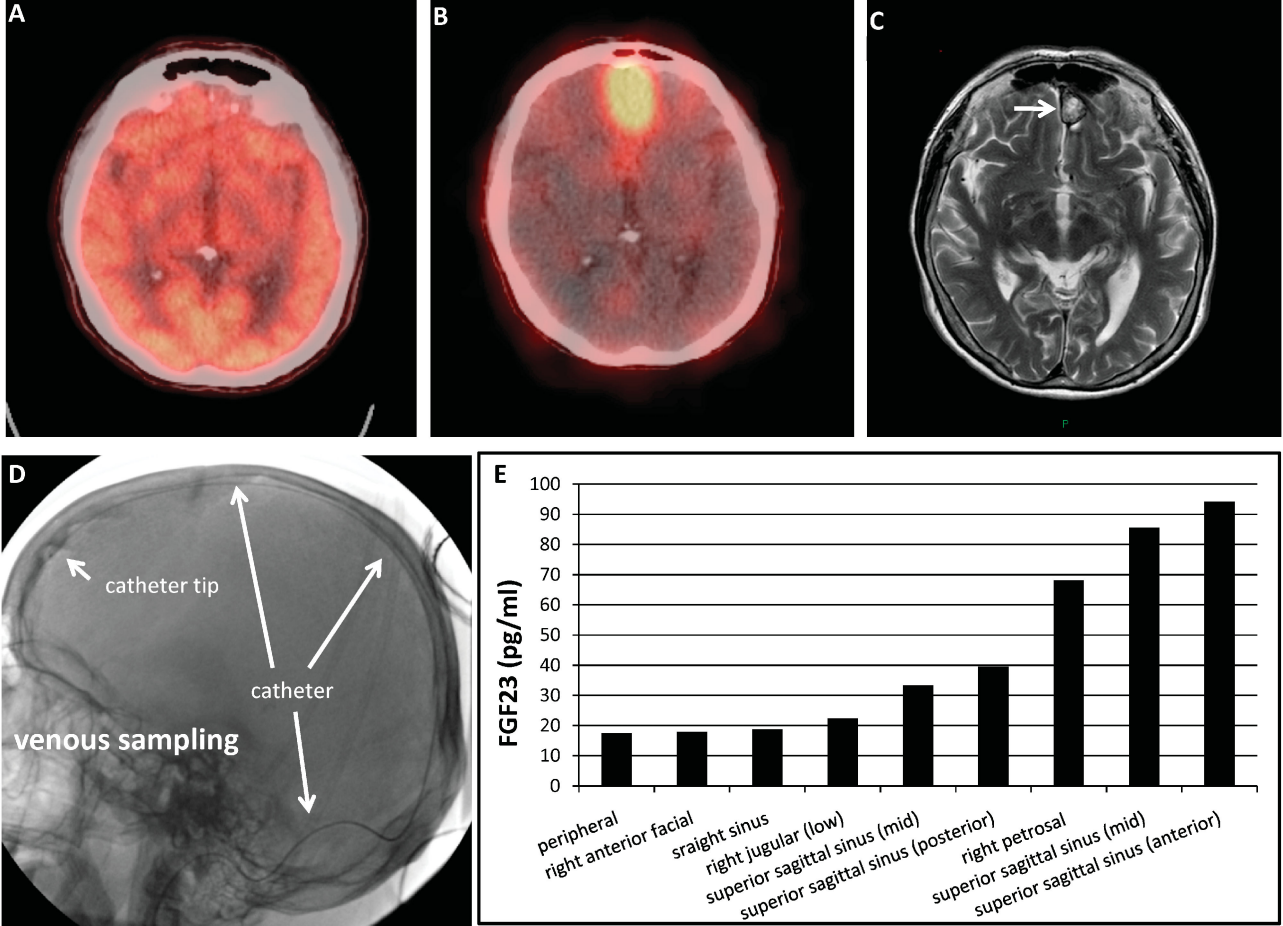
Example of a selective venous sampling that distinguished between conflicting imaging results. For a lesion that ultimately was found adjacent to the brain, FDG-PET/CT scan (*A*) was negative owing to the intense physiologic uptake of FDG by the brain. The pentetreotide/CT scan was positive (*B*), but the MRI findings (*C*) were felt to be most consistent with a meningioma, which also takes up pentetreotide. To resolve the matter, the superior sagittal sinus was catheterized from a transjugular approach (*D*), and FGF-23 concentrations were determined (*E*). The catheter and catheter tip are identified as indicated by white arrows. The results of the venous sampling were consistent with the fact that the lesion identified on pentetreotide scan and MRI was the FGF-23-secreting tumor.
